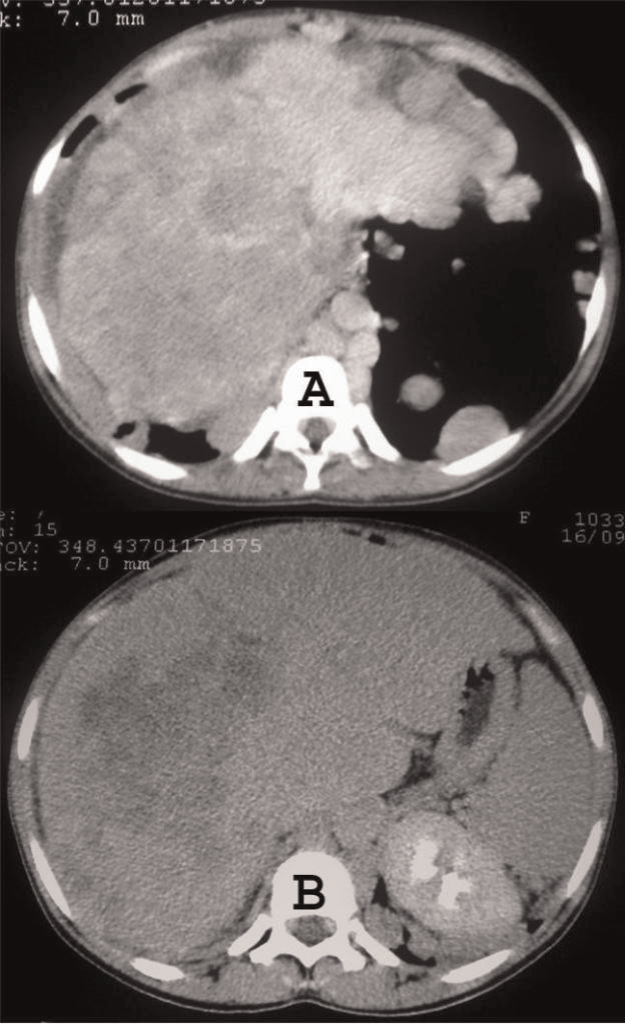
Computed tomography scans of the upper abdomen showing hepatomegaly, with an extensive ill-defined heterogeneous lesion in the hepatic parenchyma.
